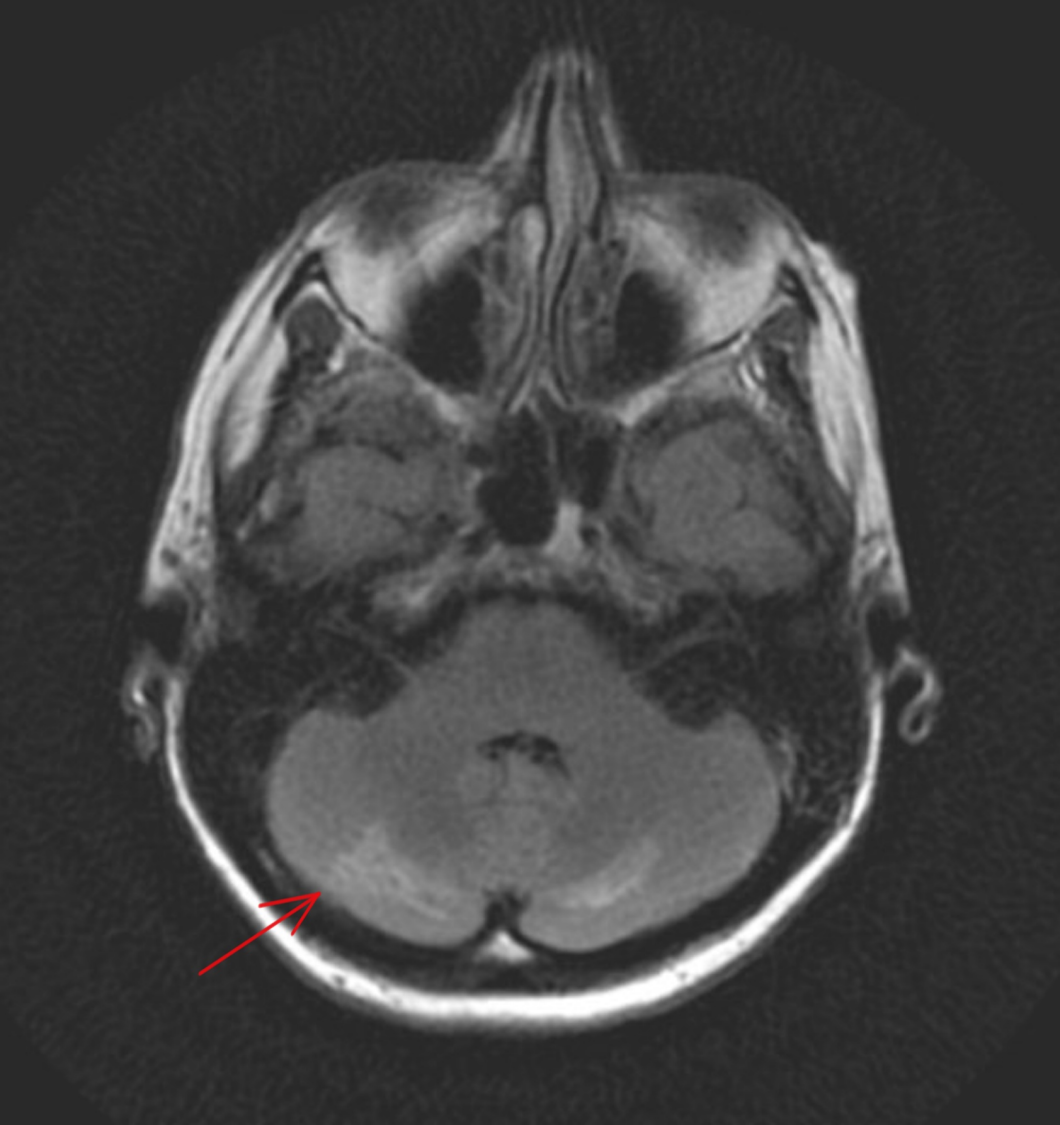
Magnetic resonance imaging (MRI) of the brain without contrast showing focal cerebellar enhancement.
